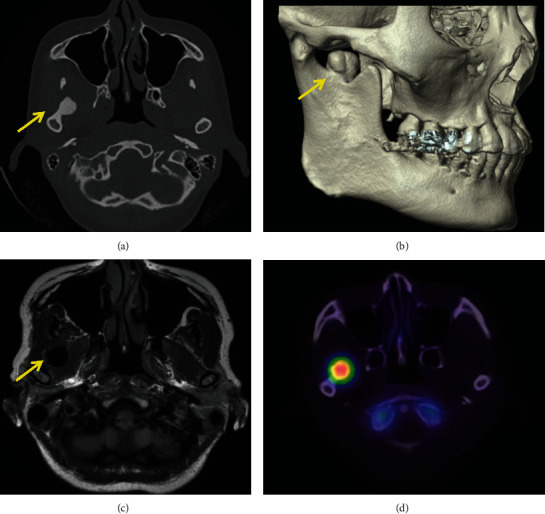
Preoperative examination. (a) Computed tomography showed a 13.3×12.8×10.0mm sized lesion showing Hounsfield units (HU) equivalent to that of the mandible, arising from the right mandibular notch. The CT values of osteoma ranged from 1480 to 1580 HU. (b) 3D-CT showed osteoma was found in the right mandibular notch. (c) Magnetic resonance imaging showed uniform hypointense trabecular bone, and there was no continuity of fat signals in the bone marrow, suggesting exclusion of the lateral pterygoid muscle because of bone lesions. (d) Radioisotope imaging showed significant uptake of Tc-99m by the osteoma in the right mandibular notch.
